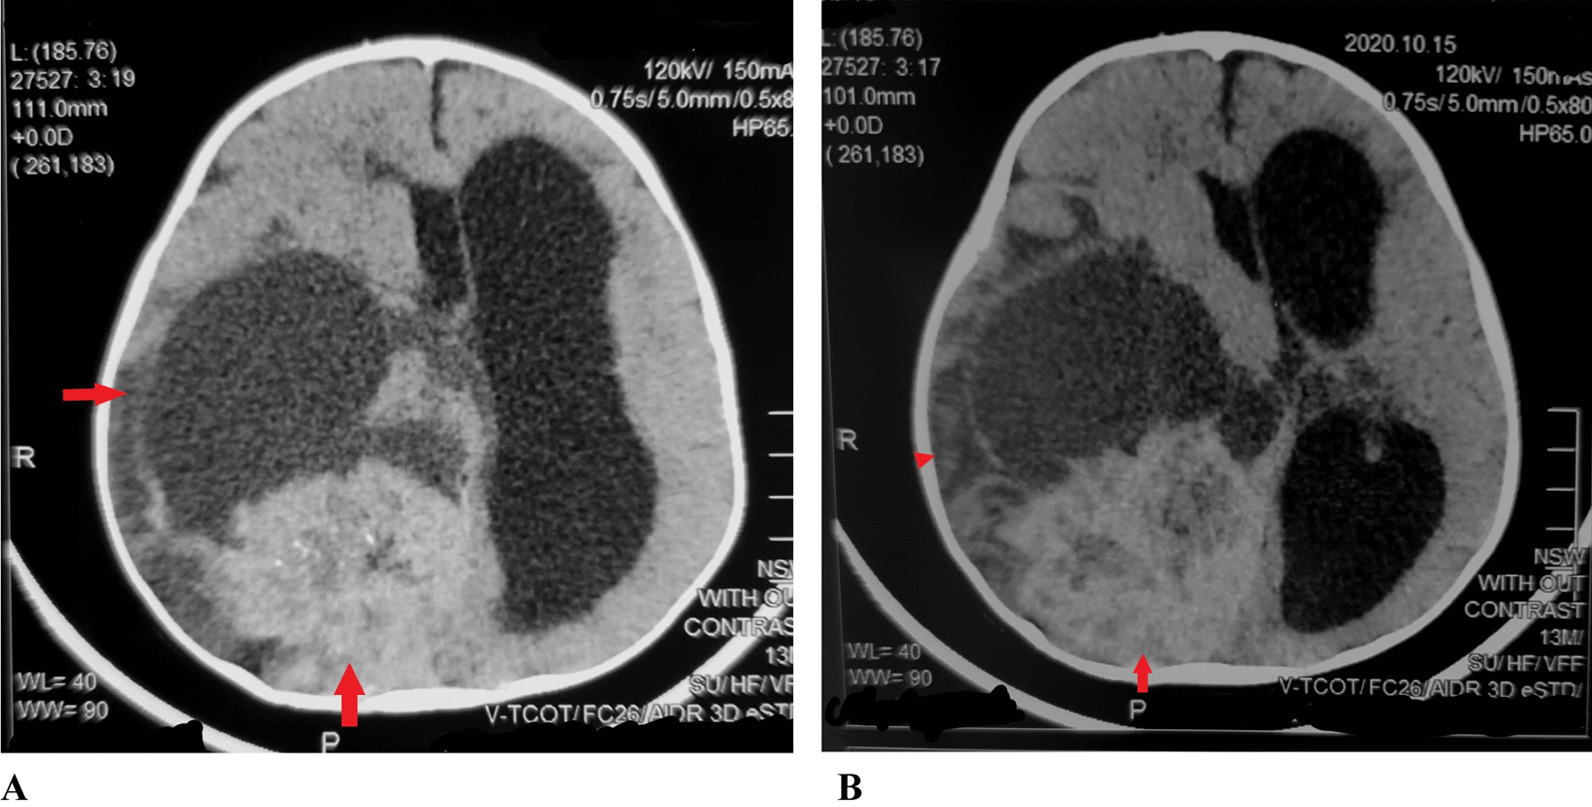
A, B Pre-operative CT without contrast: Axial images show a mixed density heterogeneously enhancing mass in the Right posterior parieto temporo occipital locations (red arrows)
